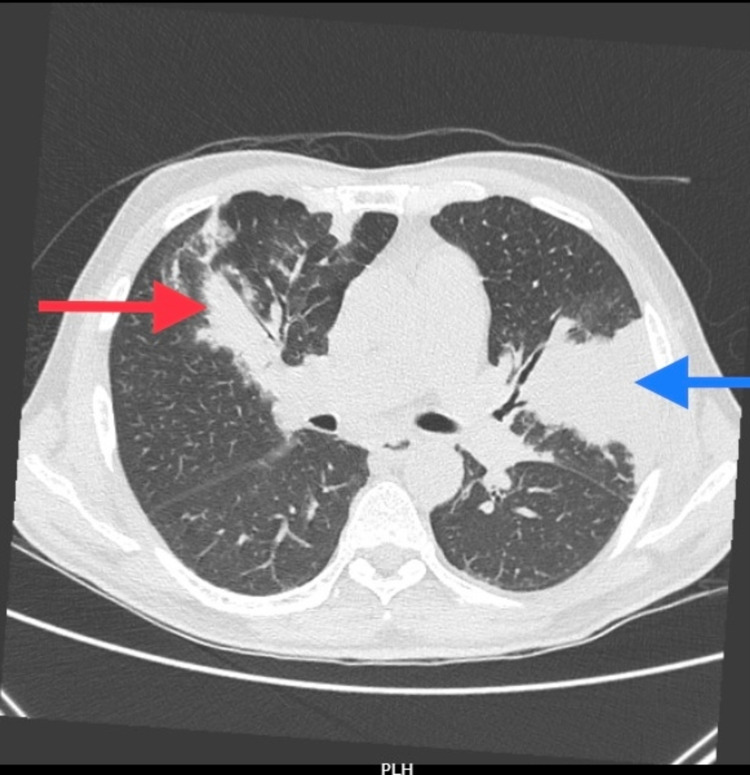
CT chest showing a consolidation involving the left lingular segment (blue arrow) along with peri-bronchial consolidation in the anterior segment of the right upper lobe (red arrow). CT - computed tomography
